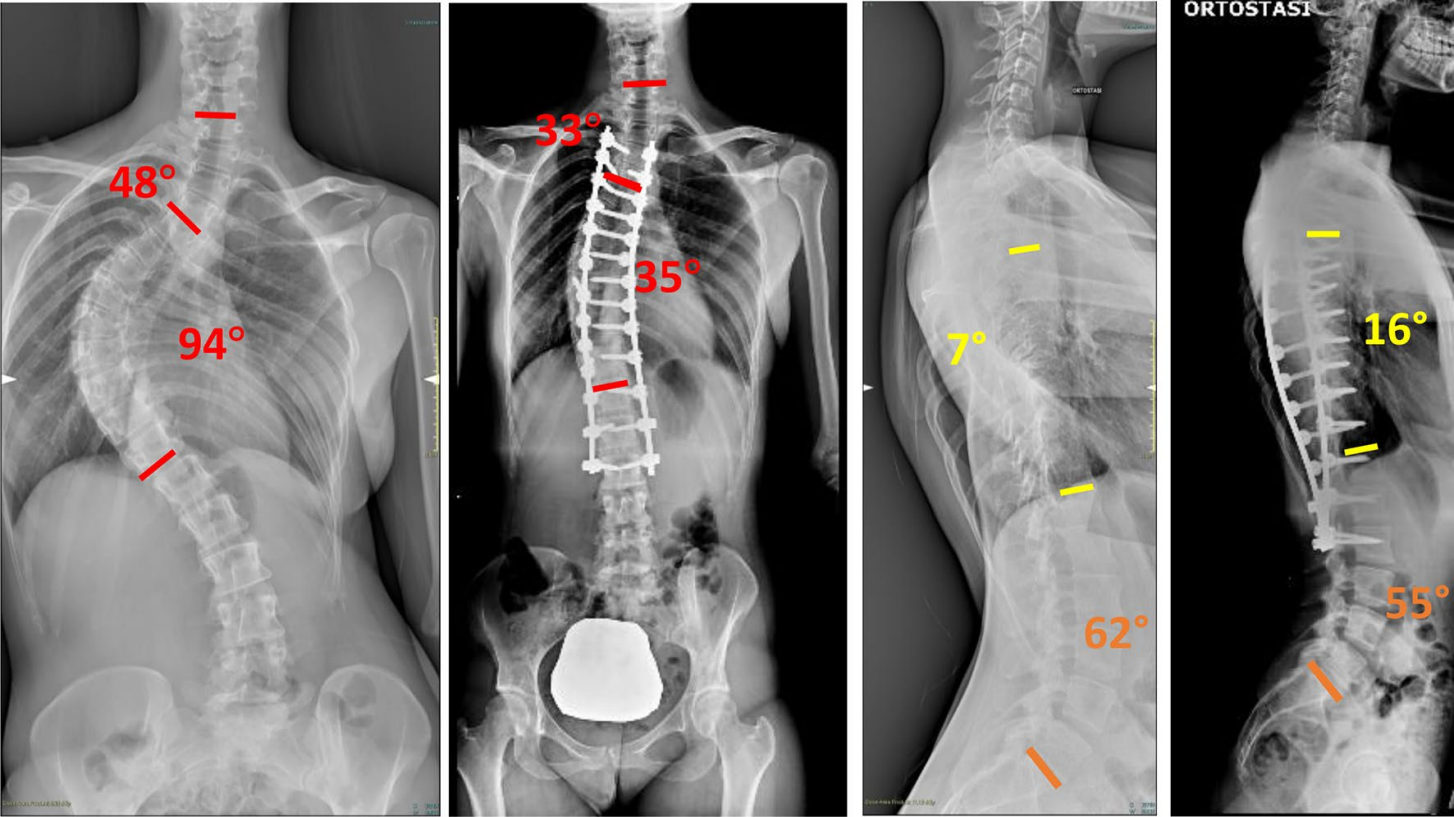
Case 4, a 18 years old female with a Lenke 2 curve, treated with a T3–L3 fusion; pre- and post-operative X-rays
